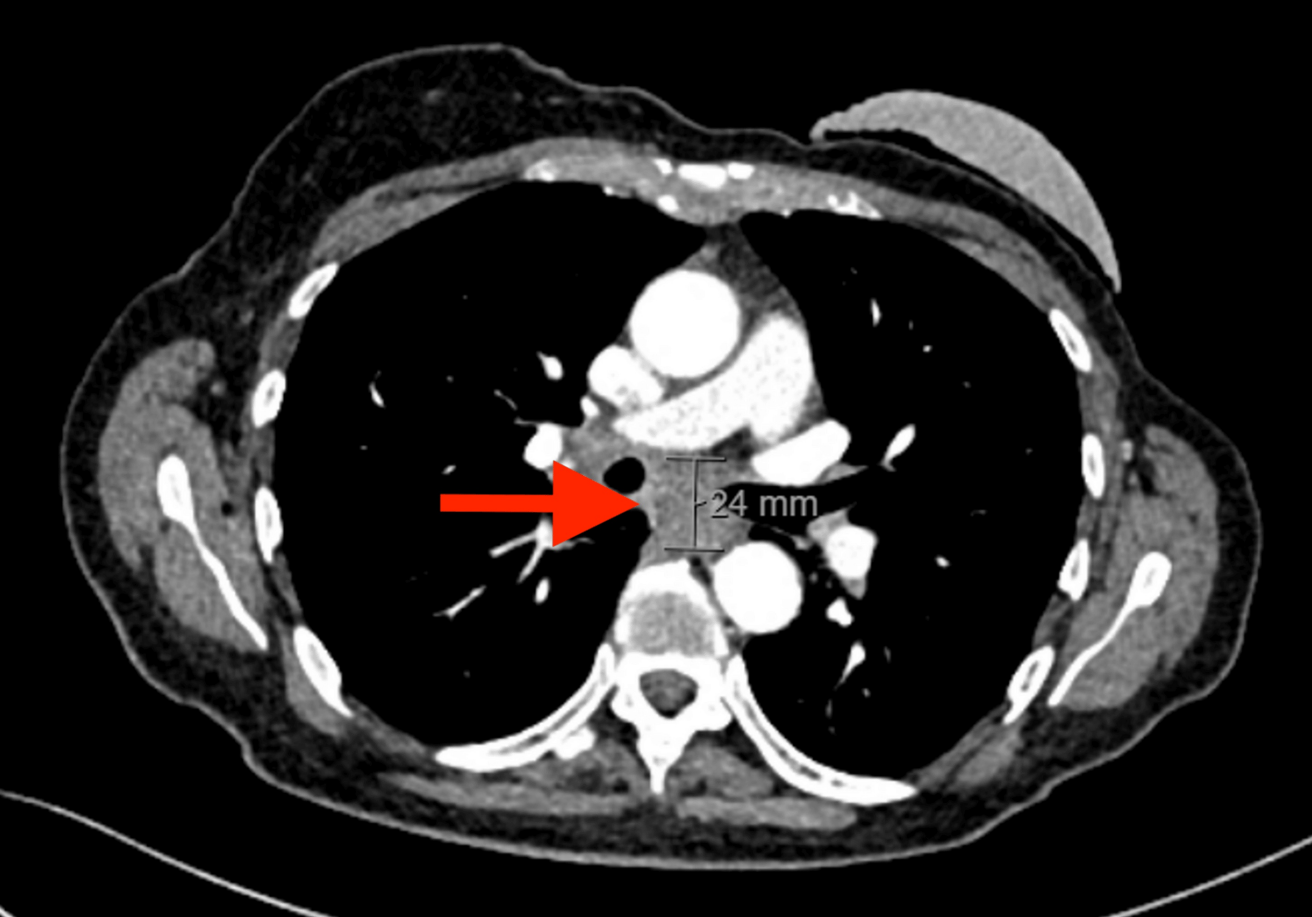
CT thorax with contrast revealed a peri-bronchial mediastinal soft tissue lesion causing stenosis in the middle esophagus (red arrow). CT: computed tomography.
